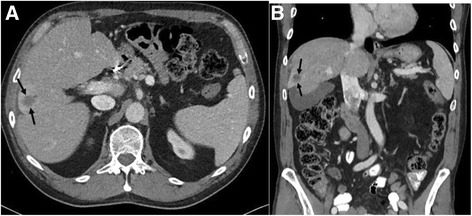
a Axial and b coronal post-contrast CT images through the abdomen after completing 13 cycles of immunotherapy and despite being off treatment for 4 months secondary to hepatitis again demonstrate the index metastatic lesion (*arrows*) within the right hepatic lobe with continuing decrease in size
